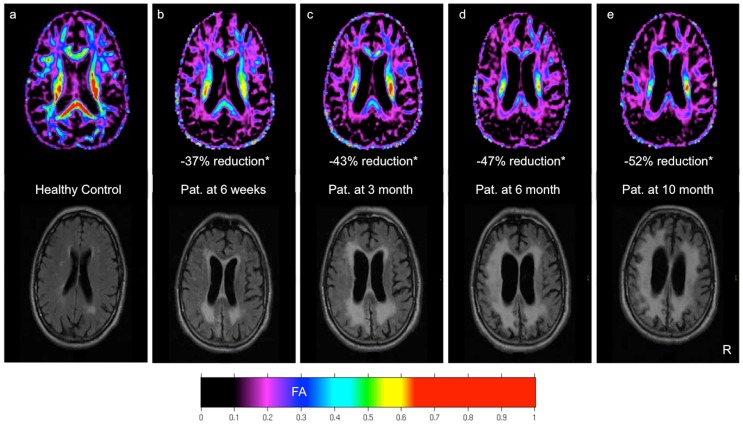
Color-coded FA map and FLAIR MRI of a 78 y old healthy control (a). DTI and MRI of the 76(b–e). * = Reduction of mean FA of the whole white matter (WWM) relative to the mean WWM FA of ten age-matched healthy control subjects.
